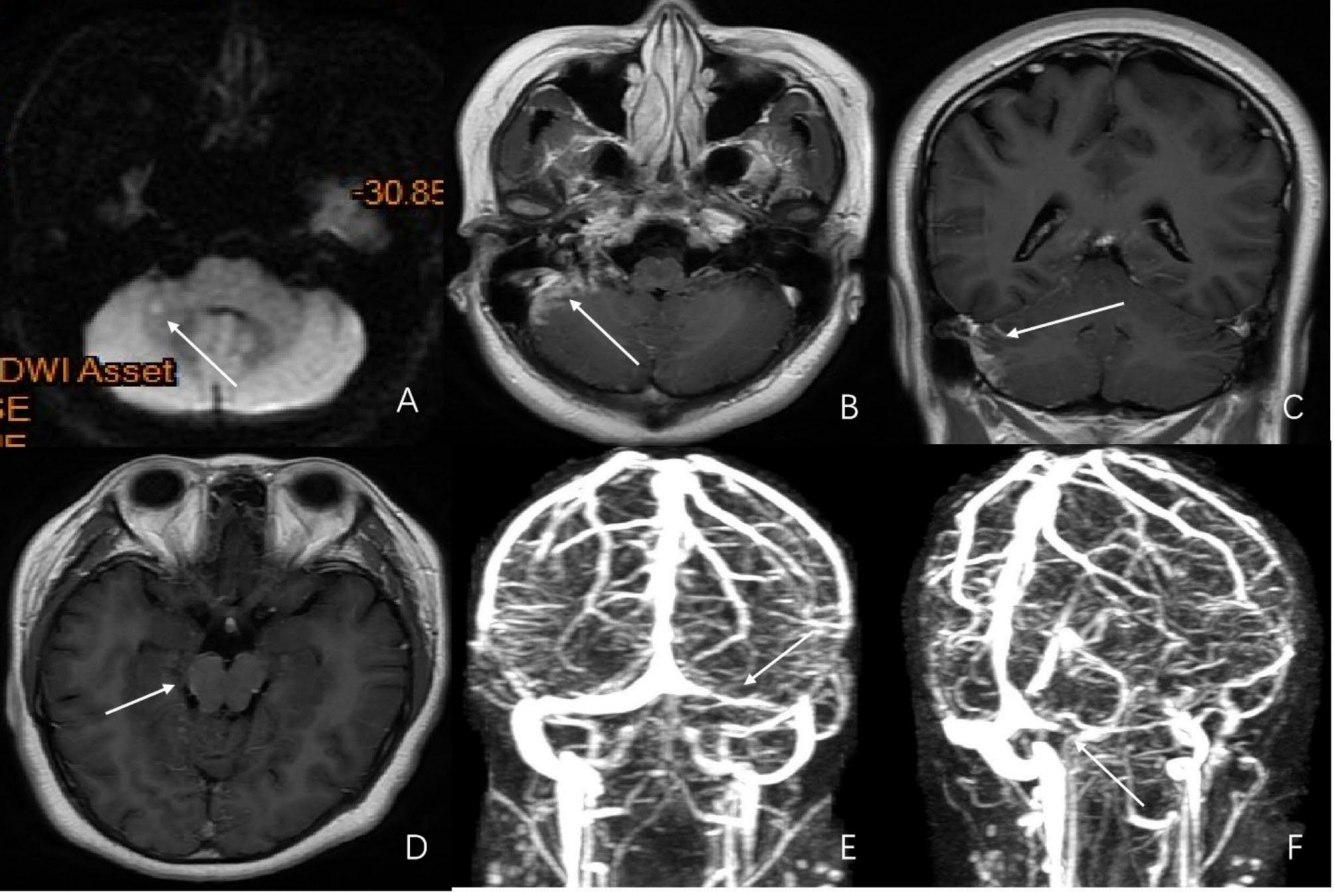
Radiographic findings of the patient in case 3. A: Brain plain MRI DWI sequence shows high-signal intensity nodules in the right cerebellum. B, C, and D: Post-contrast MRI T1WI (axial and coronal) shows patchy enhancement around the right cerebellum near the right sigmoid sinus area and enhancement of pia mater of the basal cistern around the midbrain. E and F: Post-contrast MRI T1WI shows filling defects in the left transverse sinus and sigmoid sinus
